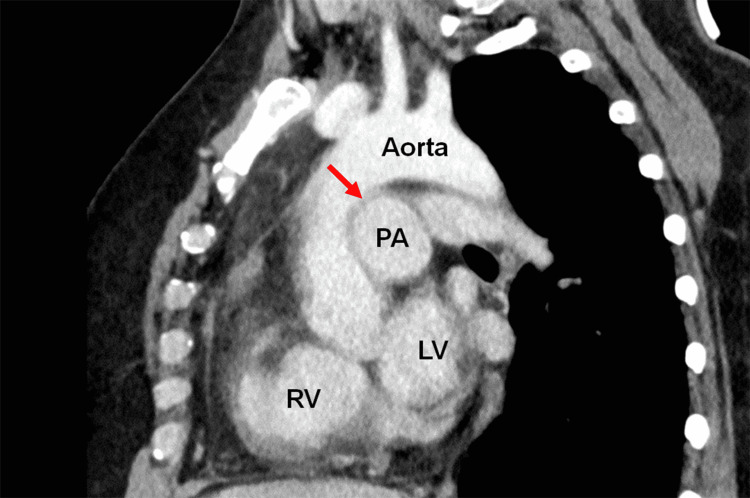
Computed tomography (CT) scan of the chest The sagittal CT image shows dilation of the main pulmonary artery and enlargement of the right ventricle, consistent with typical idiopathic pulmonary arterial hypertension findings. Dilation of the pulmonary artery results in the narrowing of the space between the pulmonary artery and aorta (red arrow) and causes compression of the recurrent laryngeal nerve. LV: left ventricle; PA: pulmonary artery; RV: right ventricle
